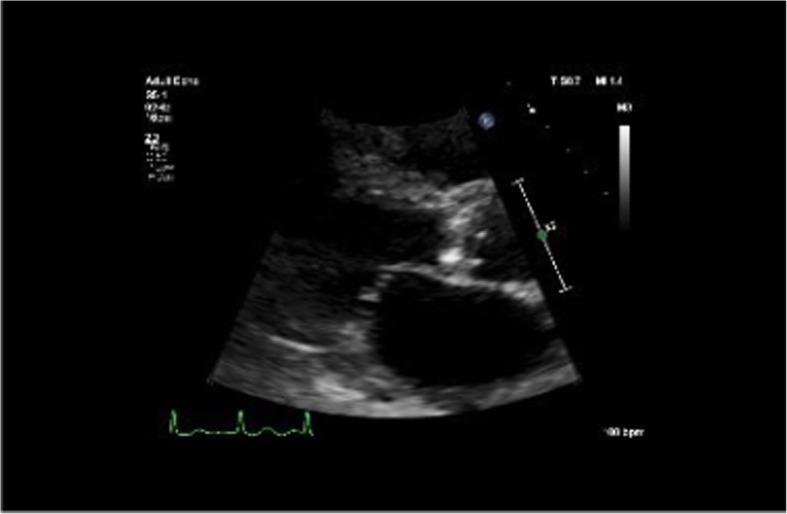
Parasternal long-axis view on 2D transthoracic echocardiogram upon patient's initial presentation, demonstrating normal left ventricular systolic function and severe aortic stenosis with no indications of vegetations or infectious processes
